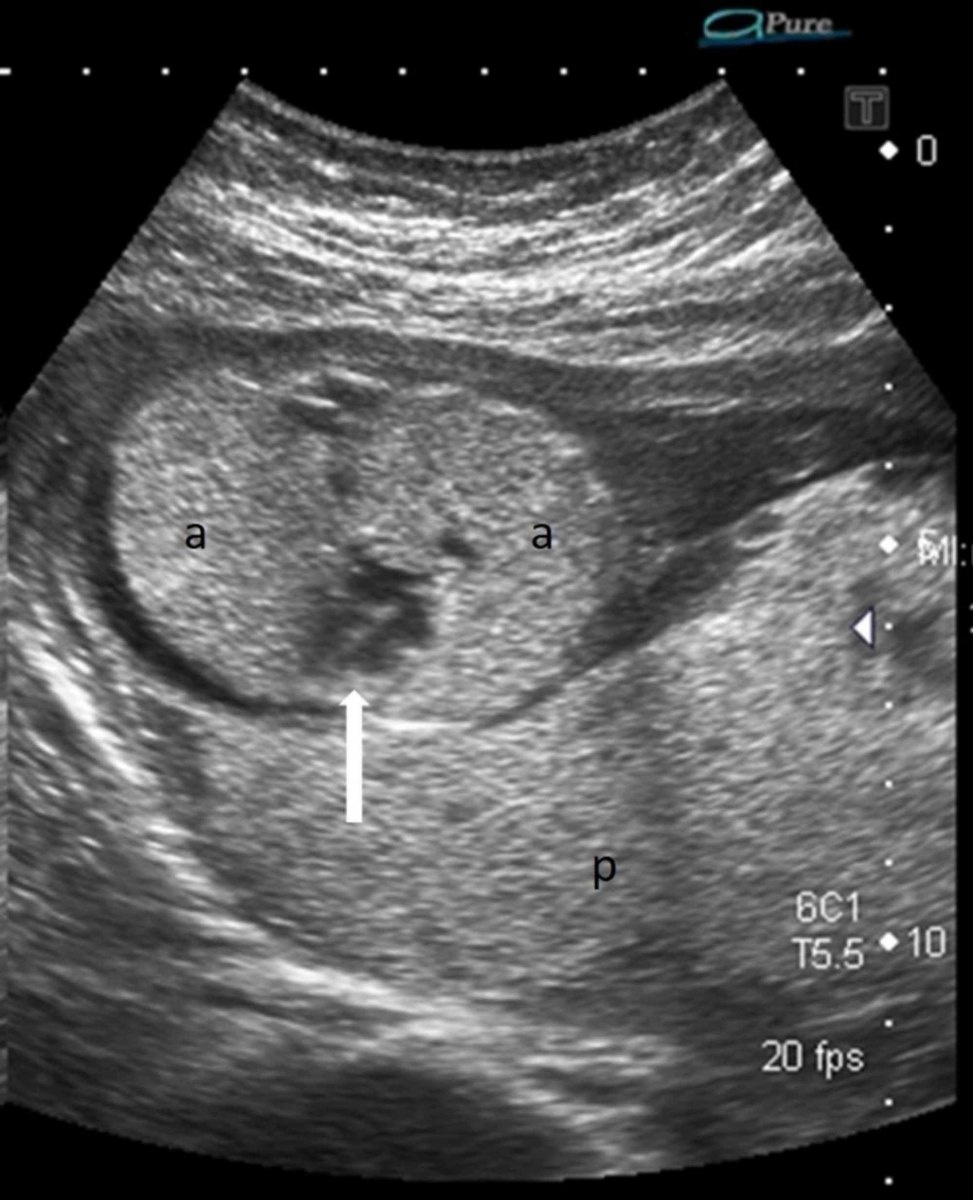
Axial ultrasound of the fetal thorax shows bilateral enlarged echogenic lungs (a) and the centrally placed heart (solid white arrow) Echogenic normal placenta (p) is seen posteriorly; oligohydroamnios is also noted.
